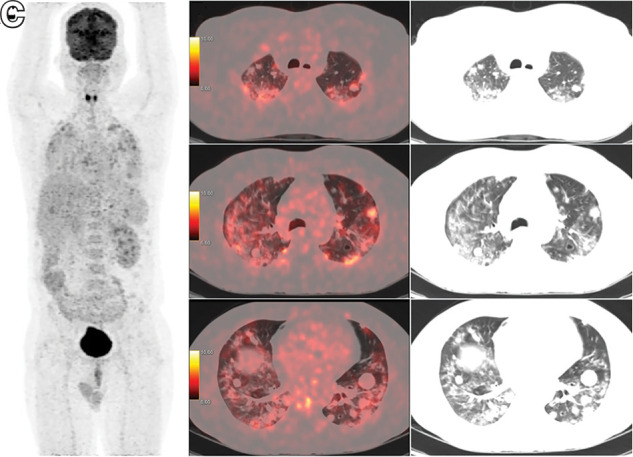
^18^F-FDG PET/CT whole-body maximum-intensity-projection image (A), axial PET/CT images (B), and corresponding CT images (C) showing ^18^F-FDG–avid diffuse ground-glass opacities/patchy consolidatory changes bilaterally in lung fields from apex to base, obscuring details of metastatic lesions (SUV_max_ of hottest nodule, 7.8; SUV_max_ of ground-glass opacities, 7.3).
